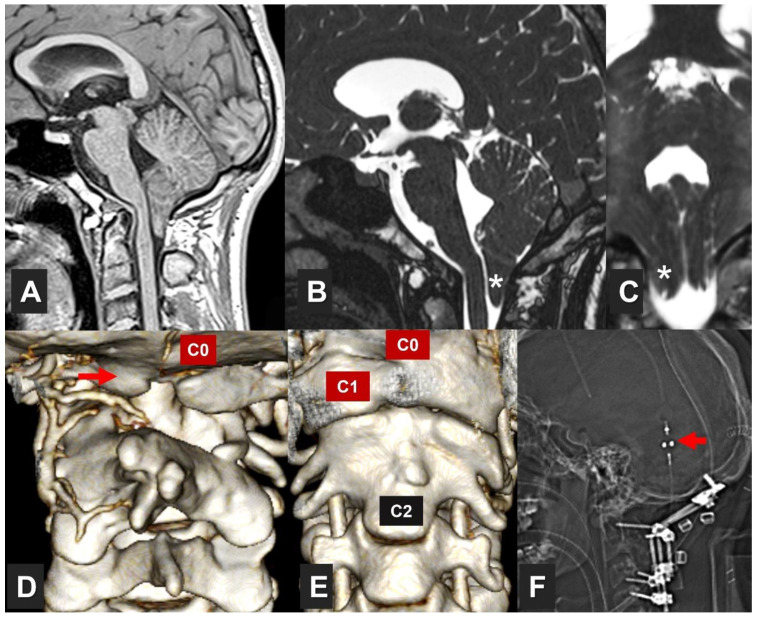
Patient with a complex craniovertebral junction (CVJ) malformation. This 17-year-old male with a VACTERL association (https://rarediseases.info.nih.gov/diseases/5443/vacterl-association) (accessed on 5 October 2023)—a non-random association of congenital disabilities that affects multiple parts of the body—was referred to our institution due to a Chiari malformation type 1 (CM1) detected as the patient presented recurrent episodes of severe occipital headaches. The neurological examination was normal. The patient had CM1 with an asymmetrical tonsillar descent 11 mm below the McRae line. (A) T1*weighted sagittal midline image; (B) T2*weighted sagittal, and (C) T2*weighted coronal slices; (D,E) three-dimensional CT reconstruction of the CVJ. White asterisks in (B,C) indicate the right cerebellar tonsil. The patient presented complete assimilation of the anterior and posterior C1 arches with an associated C2–C3 fusion and partial agenesis of the right C2 lamina. C0: posterior part of the foramen magnum. C1 labels the right lateral mass of the atlas. The red arrow in (D) shows the assimilated posterior C1 arch. Arrow in (F) indicates the valve (Polaris^®^ adjustable valve, Sophysa, Orsay, France) of a ventriculoperitoneal shunt implanted one month before because of hydrocephalus. This patient was treated with halo-vest stabilization for three weeks, followed by a one-step surgical procedure in which a posterior fossa reconstruction was conducted and instrumented occipito-C4 posterior fusion with bone-bank allograft (F).
